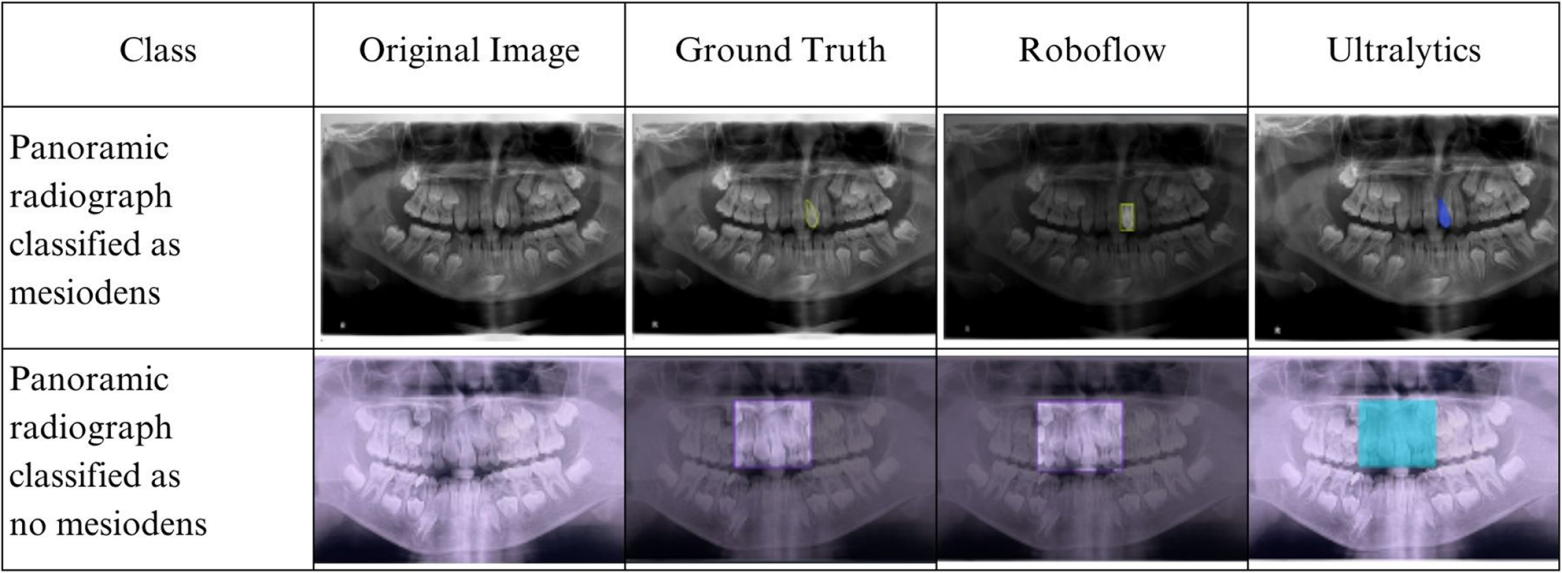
Mesiodens detection on panoramic radiograph using roboflow and ultralytics
